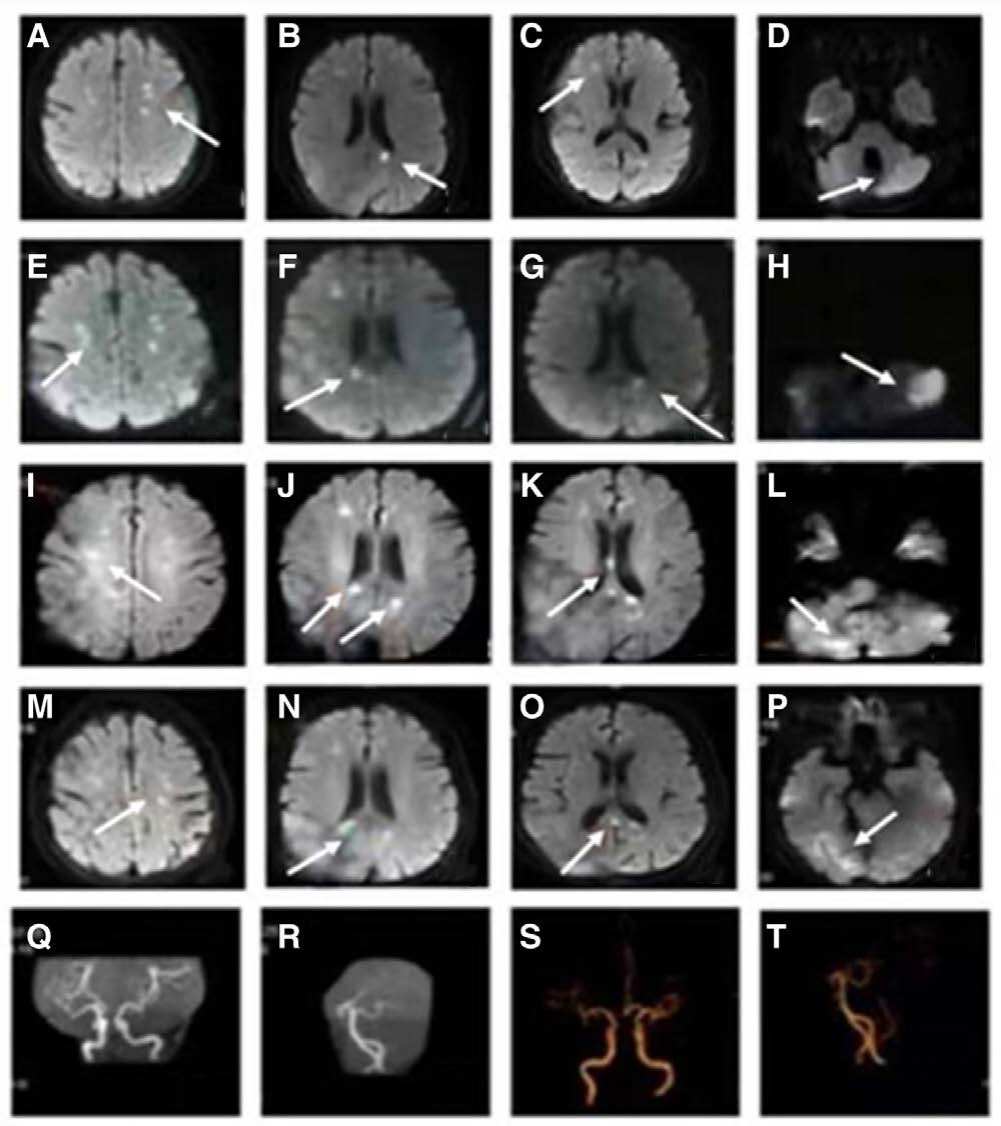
(A–D) MR imaging performance of the patient at the first visit. The arrow in (A) refers to the lesions in bilateral frontal and parietal lobes; The arrow in (B) refers to the left occipital lobe lesion; The arrow in (C) refers to the lesion of the right frontal lobe; The arrow in (D) refers to the left cerebellar lesion; (E–H) Diffusion weighted imaging (DWI) image of the patient at the second onset. (H) shows the new infarction in the left cerebellum. (I–L) DWI after the patient’s first appearance of new symptoms during hospitalization showed new infarcts in the left occipital lobe (J), corpus callosum pressure (K), and bilateral cerebellar hemispheres (L). (M–P) DWI after the second occurrence of new symptoms during hospitalization. A new infarct can be seen in the right occipital lobe at the arrow (P). (Q–T) CTA and MRA accord with arteriosclerosis.
